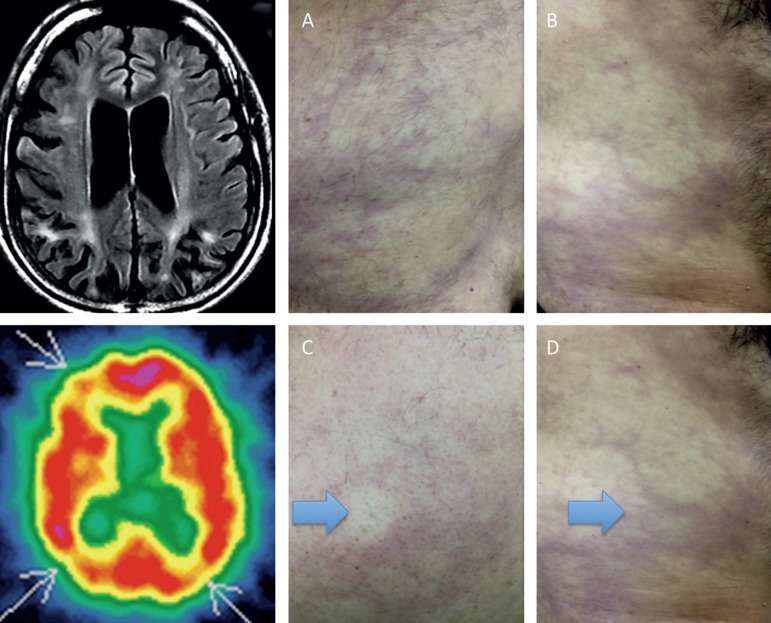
Right side with blue arrows – Livedo reticularis diffusely spread throughout the thoracic and abdominal circumference (A,B,C and D), with rapid return with finger pressure (C with blue arrow). Upper left. Axial Flair Brain MRI shows multiple areas of infarction in cortico-subcortical regions and in white matter, and diffuse brain atrophy and perfusion with 99mTc-ECD – Brain SPECT shows marked diffuse hypoperfusion in parietal and frontal lobe areas (arrows).
